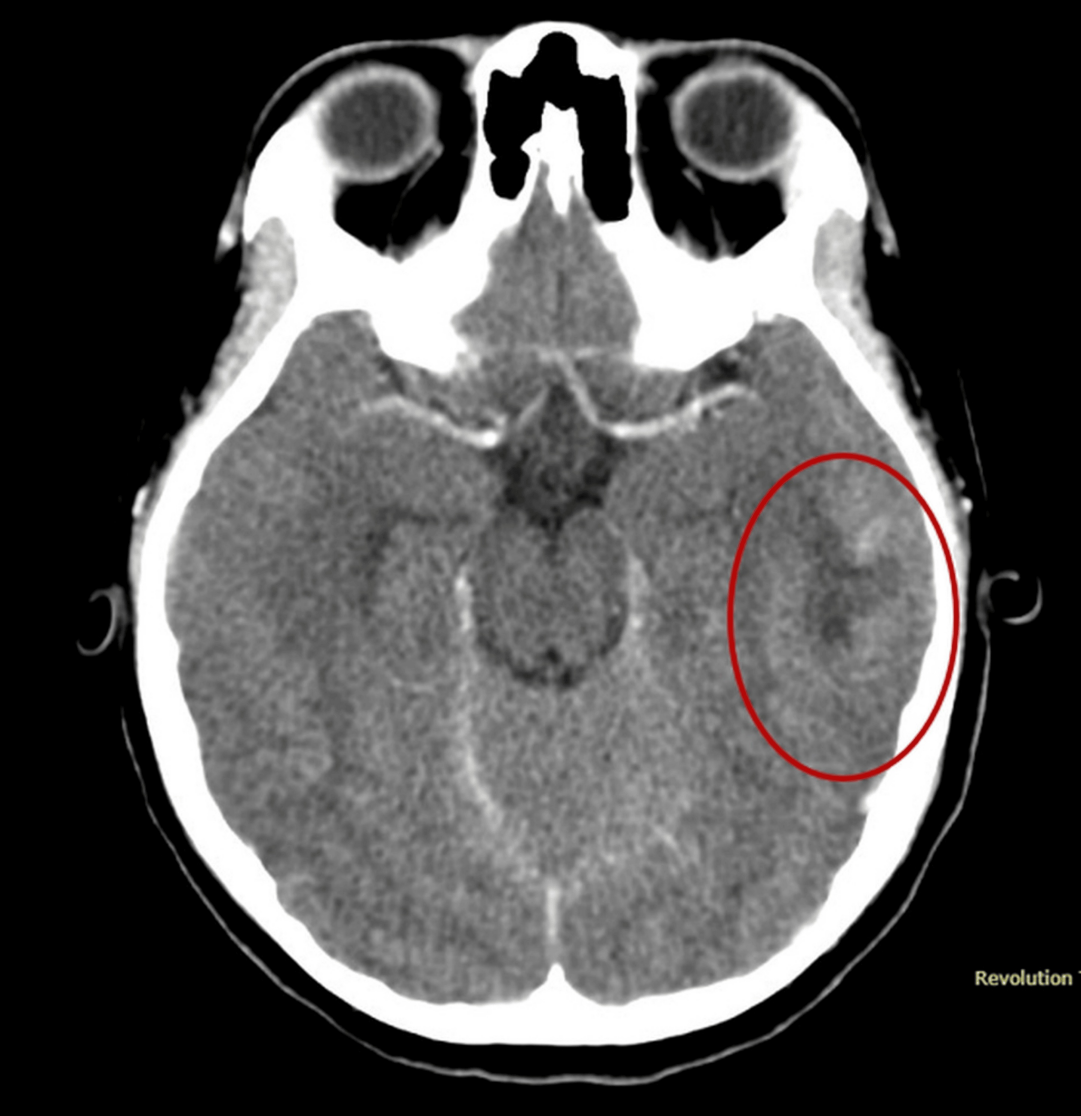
Computed tomography (CT) of the brain demonstrating cortical and subcortical infarcts in the left temporal lobe, with areas of cerebritis and small infarctions (red circle)
